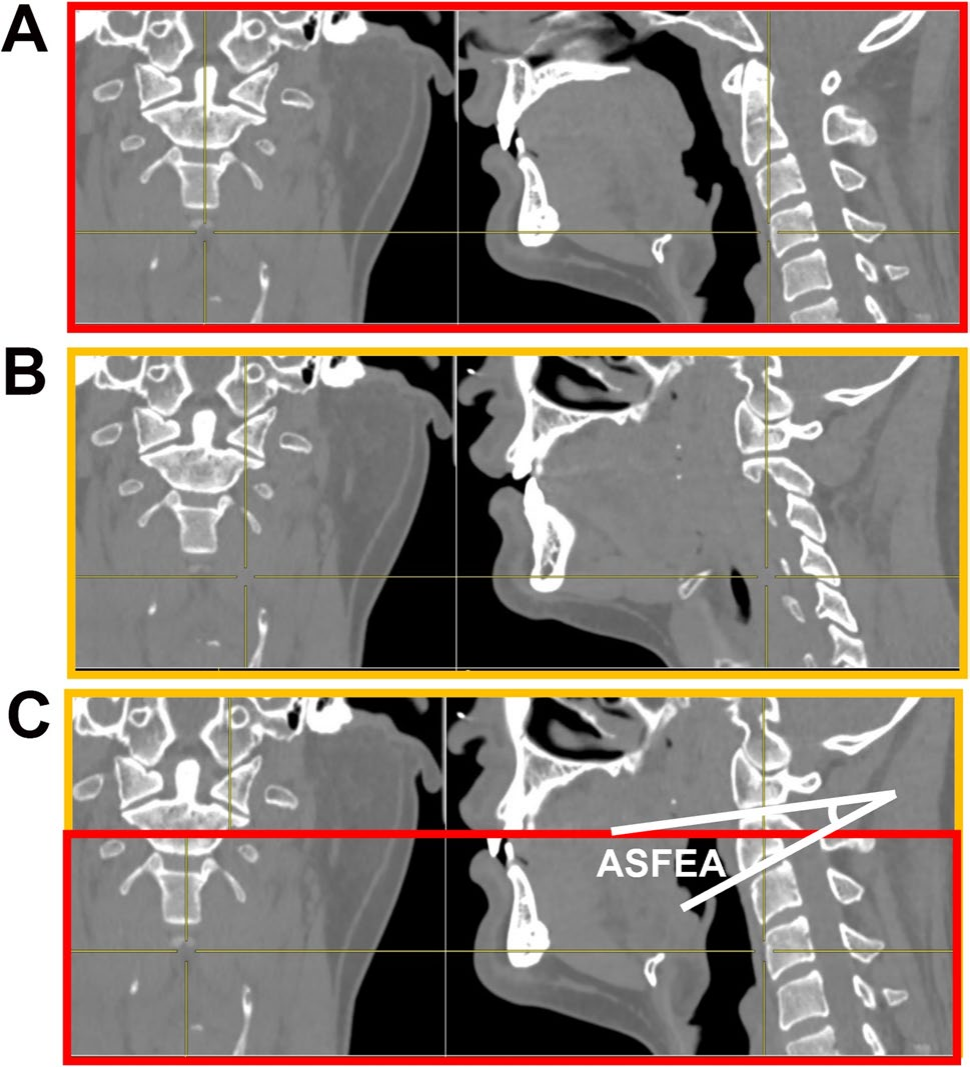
Method for ASFEA measurement. A Mid-sagittal CT reconstruction of the axis (red frame) and ASF (orange frame) (B) were obtained. C Two images were aligned, overlapped and cropped to demonstrate the axial inferior endplate and ASF simultaneously. The ASFEA was the angle between the line connecting the anterior and posterior points of the ASF and the extension line of the axial lower endplate
